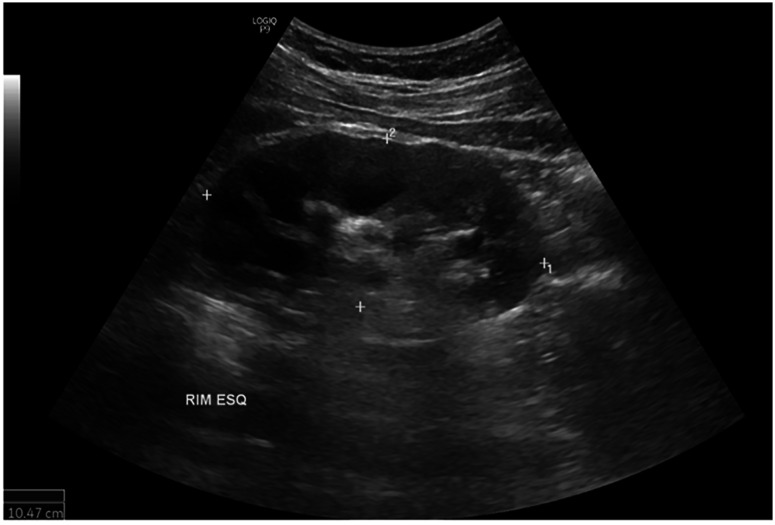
Urinary ultrasound showing multisegmental dilation of the renal calyces on the left, most prominent in the upper third, causing mild cortical thinning in this region. The findings suggest chronic multifocal infundibular stenosis associated with involvement of the collecting system.
